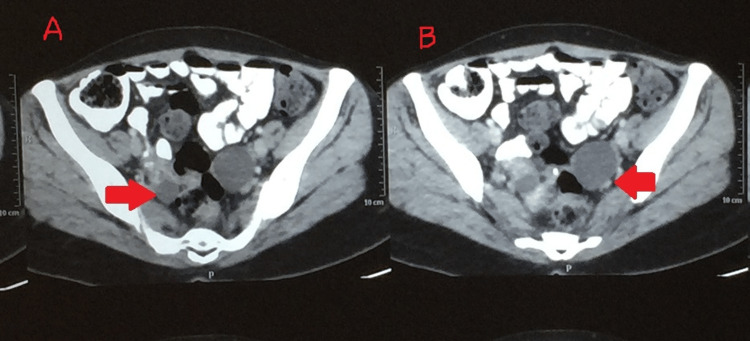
Multi-detector row computed tomography (MDCT) of the abdomen and pelvis showing bilateral cysts A) 30 mm follicular cyst in the right ovary (arrow); B) 37 mm X 31 mm cyst in the left ovary (arrow)
